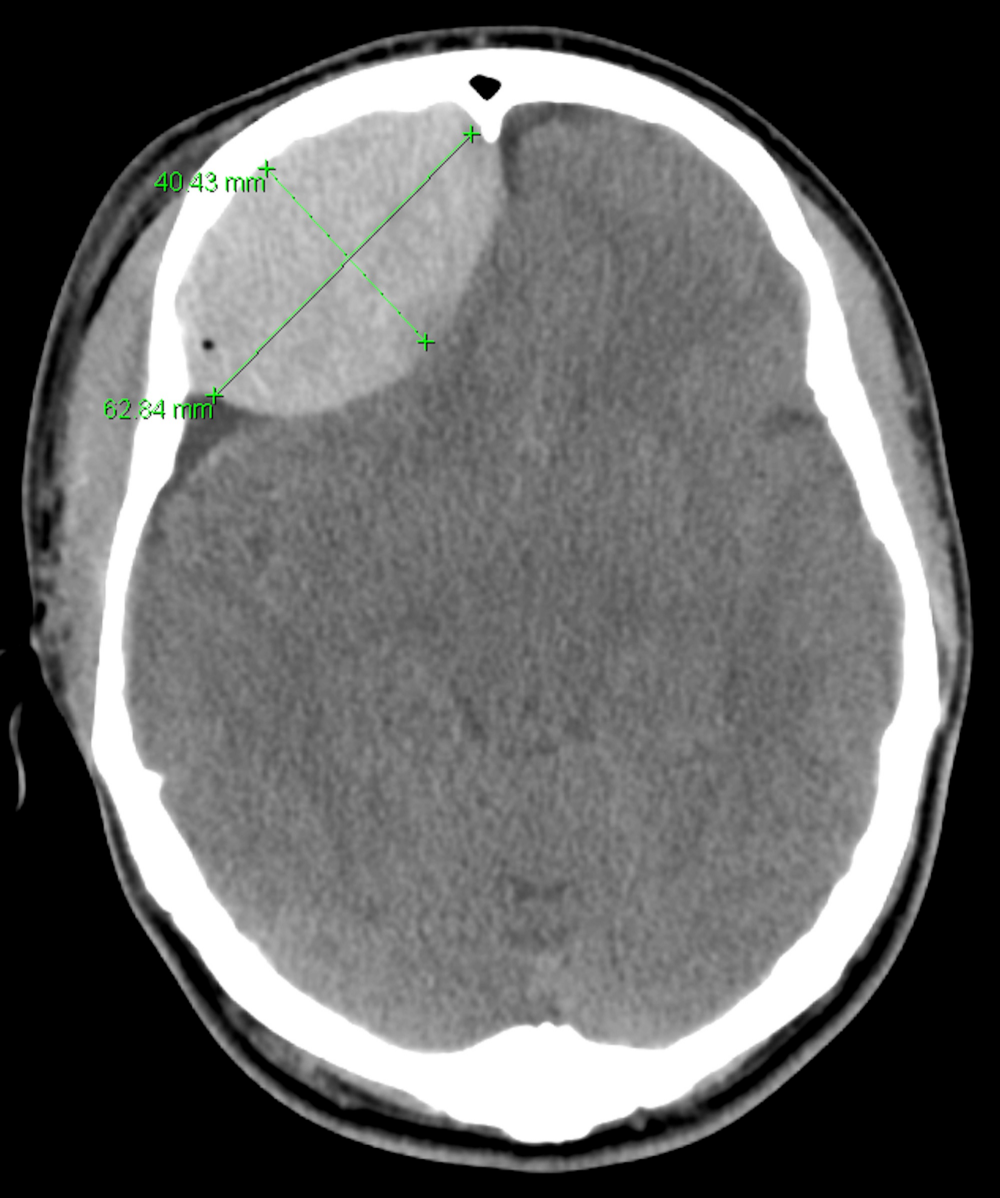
Computed tomography (CT) scan of an 18-year-old bicycle versus vehicle head-on patient Computed tomography (CT) study conducted on an 18-year-old male patient upon the immediate deterioration of the initial presenting condition (GCS 13 to GCS 5 in four hours). This image highlights the presence of a large, acute, right-sided EDH, measuring 62.84 mm by 40.43 mm, as well as a 6-mm midline shift. The patient underwent an immediate craniotomy where the entirety of the EDH was removed. Intraoperatively, a nondisplaced frontotemporal skull fracture was noted. The patient responded positively to the surgical treatment and was discharged on postoperative day four with a complete resolution of the initial and developed conditions.
